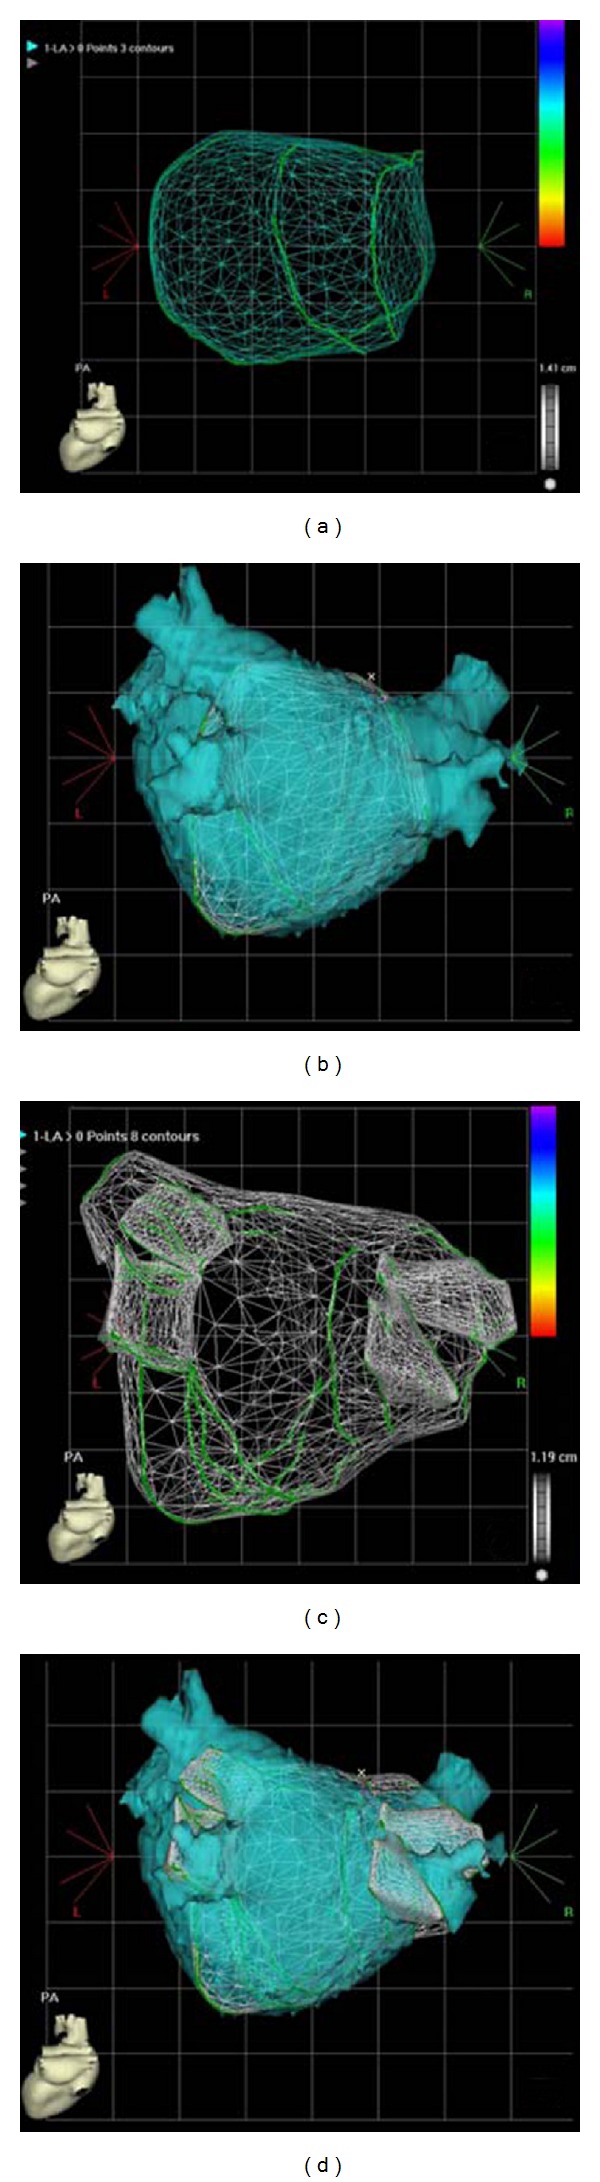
Three-dimensional ultrasound reconstruction of left atrial anatomy. (a) Minimal number of three ICE fans. (b) CARTOSound merge of three ICE fans with CT reconstruction of the LA. (c) Same LA with additional ICE fans of the body of the LA and all PVs. (d) CT reconstruction of the LA overlying all ICE fans (no further adjustment of LA orientation has been made).
